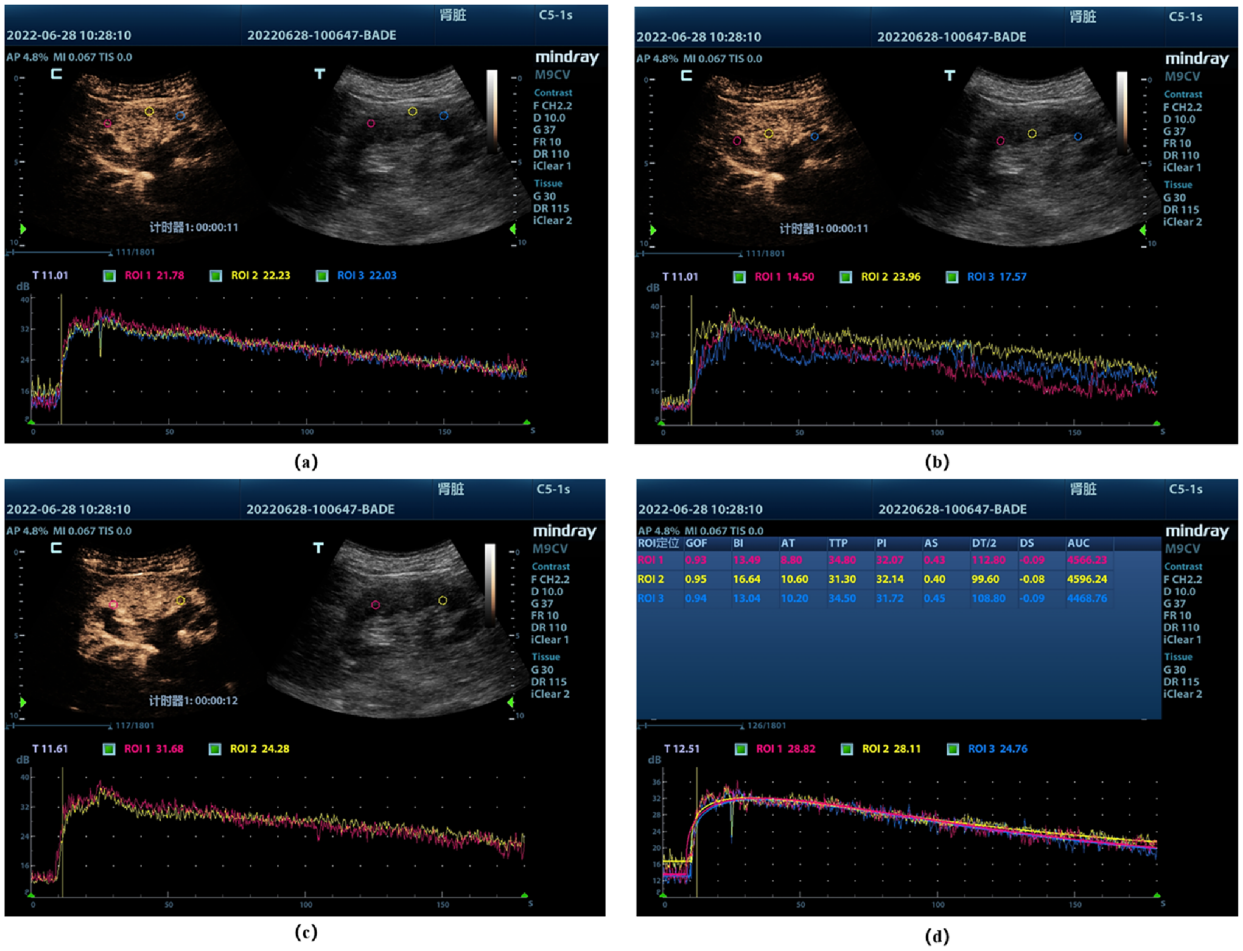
Time–intensity profiles of different sites. a Cortical sampling site and TIC, the screen shows the contrast-enhanced image (C) As well as the conventional ultrasound image (T). b Medullary sampling site and TIC. c Corticomedullary junction sampling site and TIC. d Plotting of regions of interest (ROIs) analyzed by the software, and then generating the supply curves for each ROI (lower section), these curves represent intensity as a function of time. The above images were derived from our own patients which was approved and agreed the requirement for informed consent by the institutional review board of Peking Union Medical College Hospital (approval number, I-23PJ1284)
